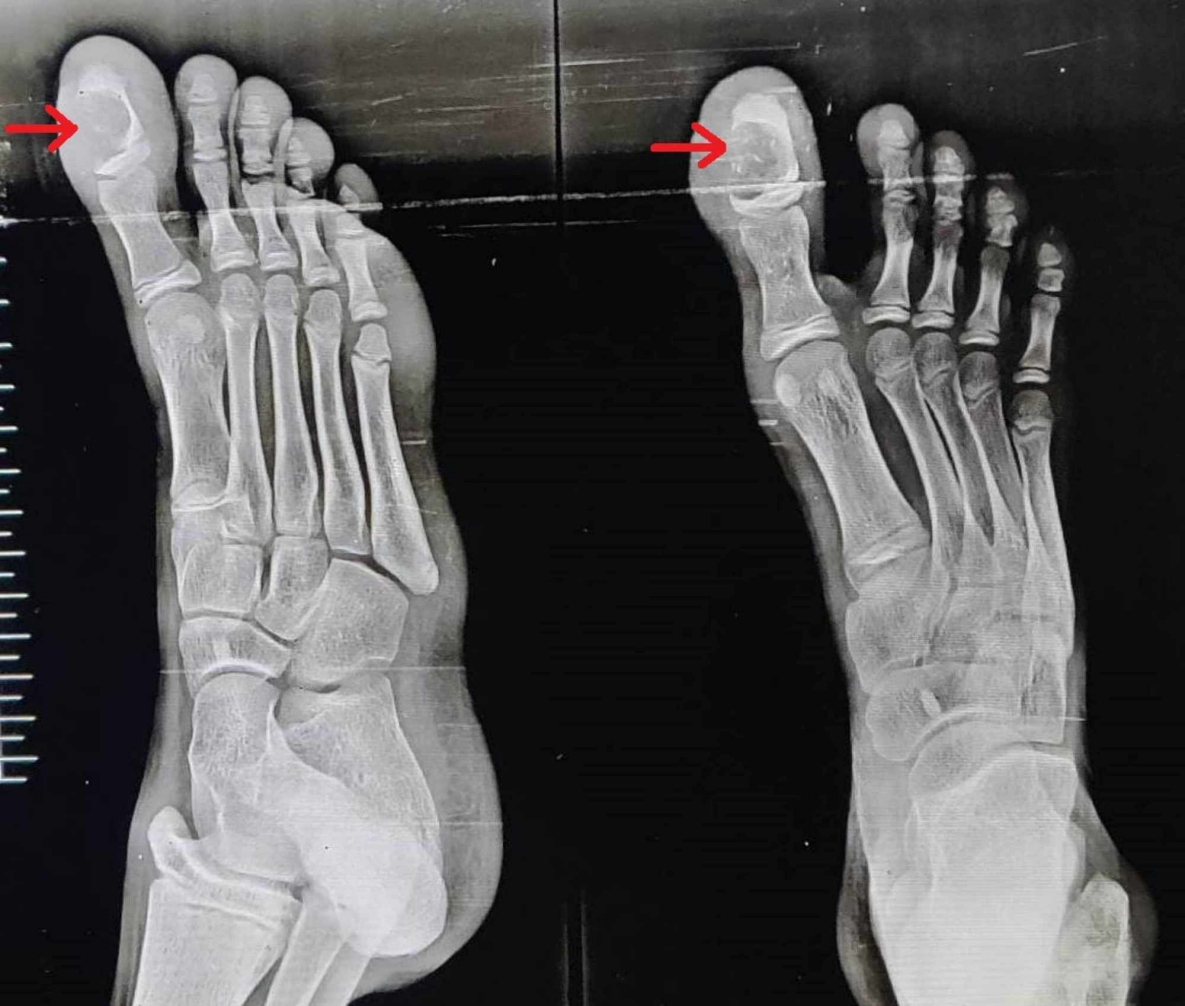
Anteroposterior and lateral view X-rays of the right foot The image shows the lytic lesion in the great toe
